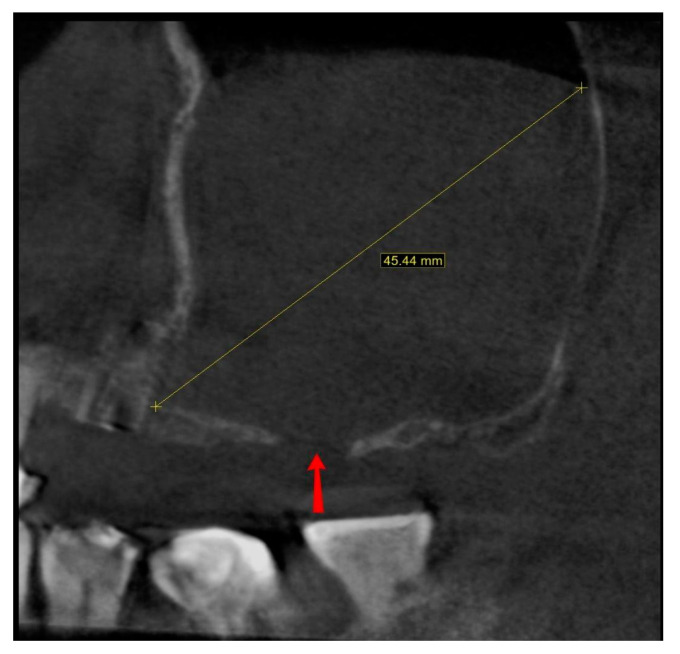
Screenshot from the pre-op CBCT scan showing maximal retention cyst’s diameter (45.44 mm; yellow line). Also, the past oroantral communication window is visible (red arrow).
